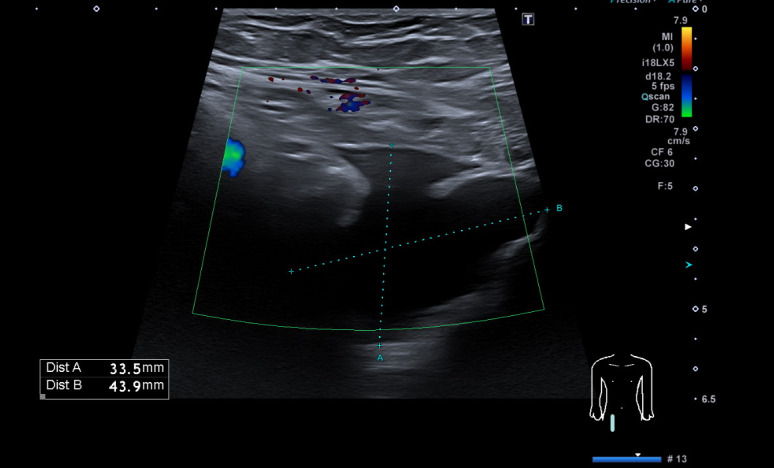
Radiology performed ultrasound of the pelvis demonstrating free fluid in the right inguinal region. No enhancing flow pattern is seen on colour Doppler.
